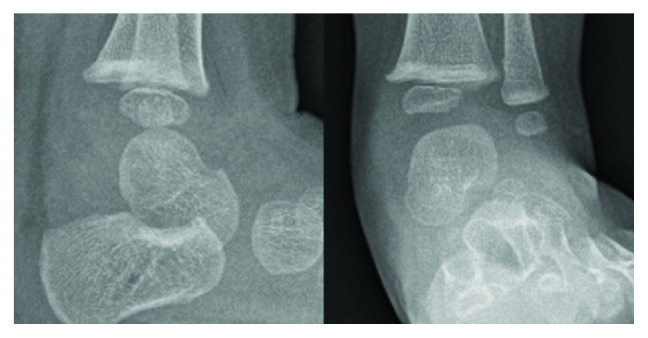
Lateral and AP plain radiograph of the ankle at presentation.
